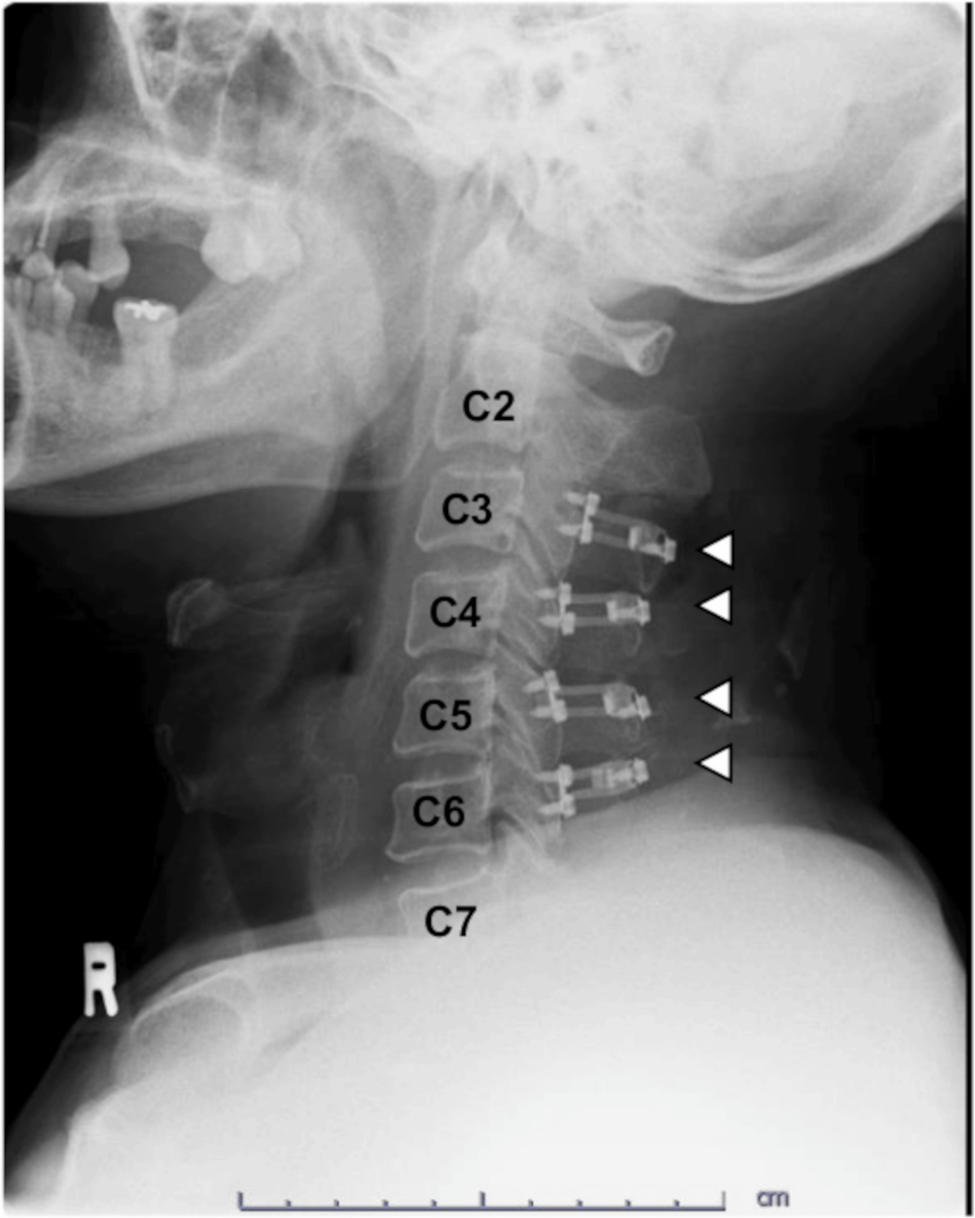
Postoperative cervical lateral X-ray image Cervical laminoplasty was performed from C3 to C6 levels. Spacers (white arrowheads) were observed.
